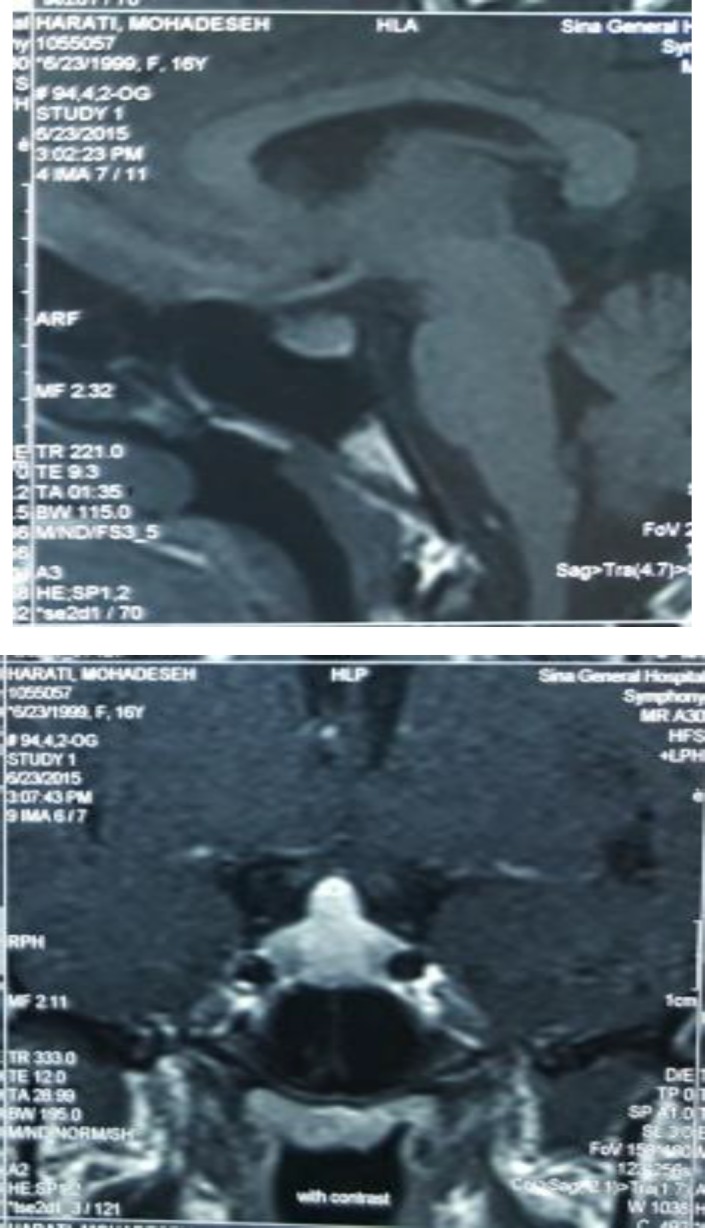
First MRI before treatment showed a 17×12×11 mm pituitary mass
